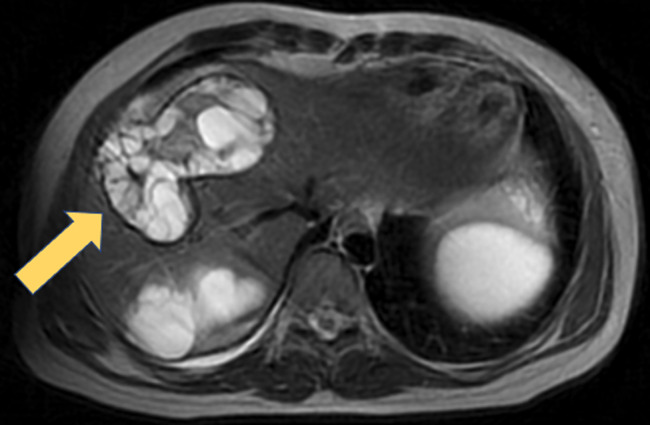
T2 sequence of magnetic resonance imaging of the upper abdomen; within the liver, there are multiple cysts, including several smaller cysts inside them (arrow); hepatomegaly is also noted; other cysts, containing smaller cysts were also noted in the peritoneal cavity between the intestinal loops, the parametrium and the ovaries
